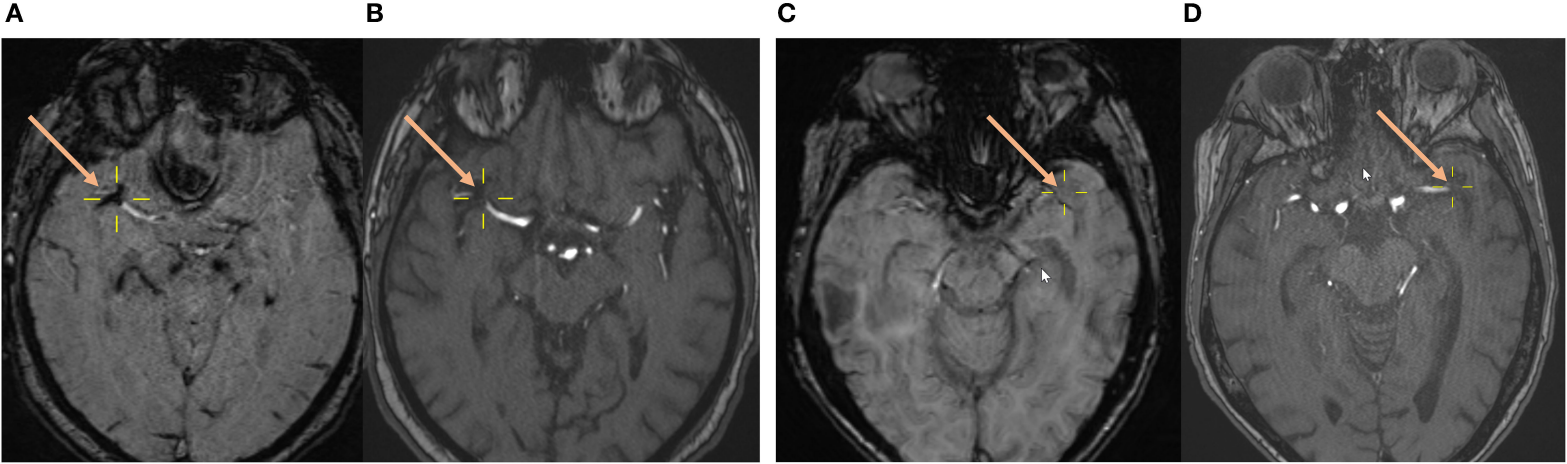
Assessment of the susceptibility vessel sign status on baseline brain MRI. (A,B) A 74-year-old male patient with AIS and visible SVS as a circumscribed signal loss on the SWI (A) with complete occlusion of the right MCA (M1 segment) on arterial TOF (B). (C,D) A 77-year-old female patient with AIS and absent SVS on the SWI (C) despite complete occlusion of the left MCA (M1 segment) on arterial TOF (D). *Yellow crosshairs* and *salmon arrows* center, respectively, point to the proximal part of the vessel occlusion on SWI and TOF. AIS, acute ischemic stroke; MCA, middle cerebral artery; SVS, susceptibility vessel sign; SWI, susceptibility-weighted imaging; TOF, time-of-flight angiography.
